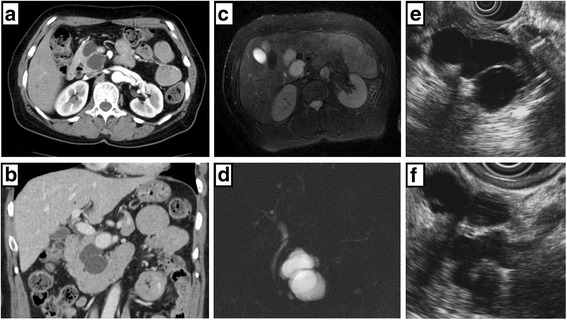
Axial (a) and coronal (b) contrast-enhanced computed tomography showing a cystic mass in the pancreatic head. Fat-suppressed T2-weighted magnetic resonance imaging (c) and magnetic resonance cholangiopancreatography (d), showing a 50 × 33-mm cystic mass, without dilation of the main pancreatic duct. Endoscopic ultrasound image, showing a 50-mm hypoechoic area in the pancreatic head (e). Mural nodules and dilation of the main pancreatic duct upstream, in the distal pancreas, were not observed (f)
